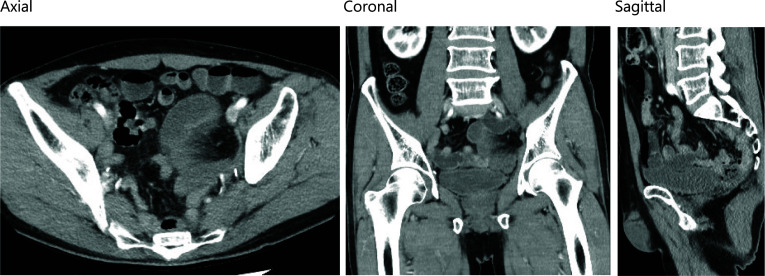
Contrast-enhanced CT scan of the case 1 showed closed-loop in the left pelvic cavity (left: axial, center: coronal, right: sagittal)
